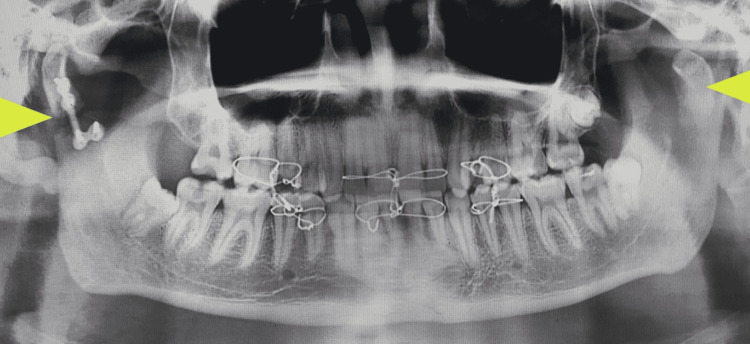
Postoperative orthopantomogram showing miniplate osteosynthesis of the right condylar fracture (arrow) and medially dislocated left condylar segment (arrow)
